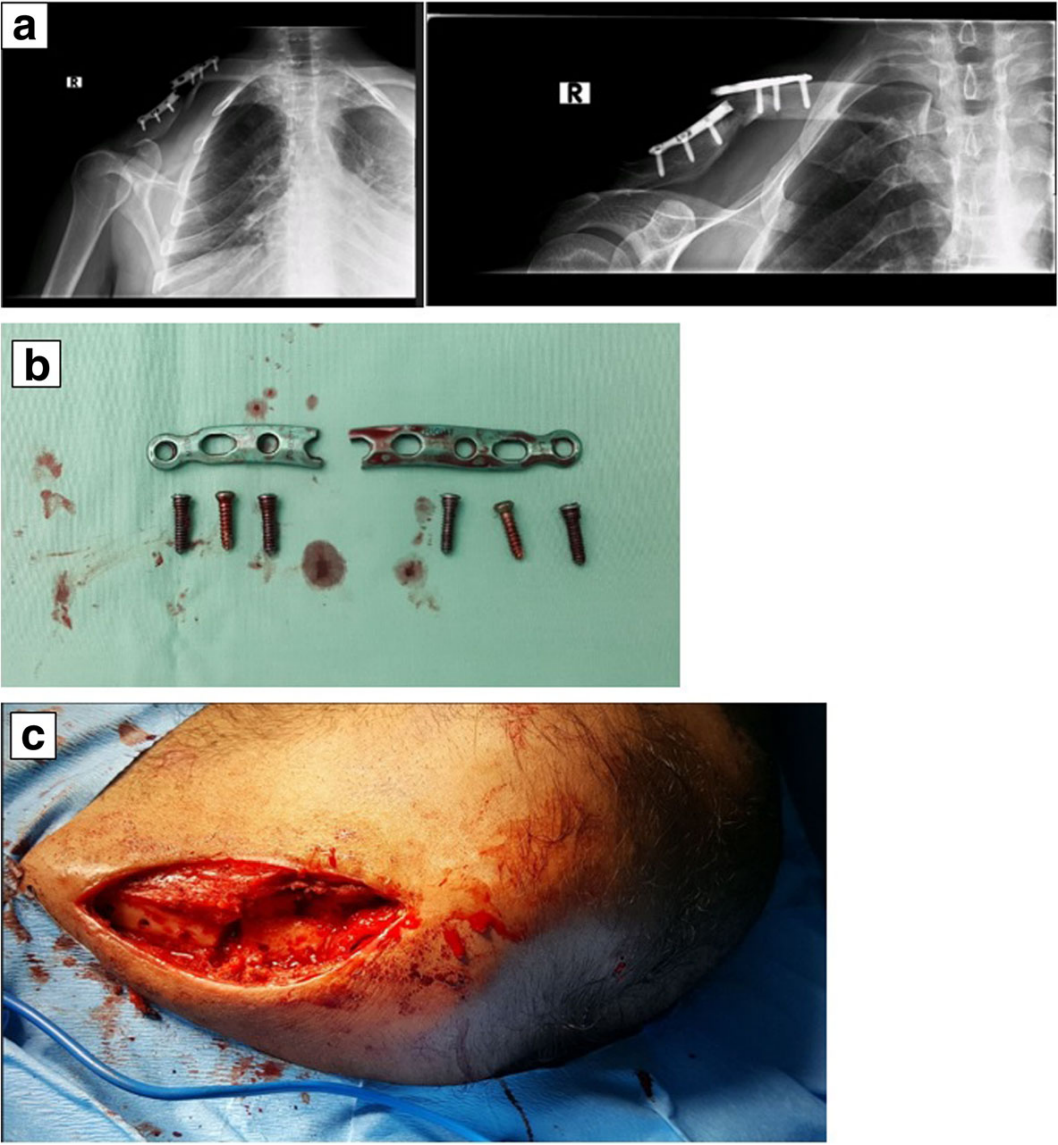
a X-ray: implant failure with plate breakage; b plate breakage; c an image of the failure area
